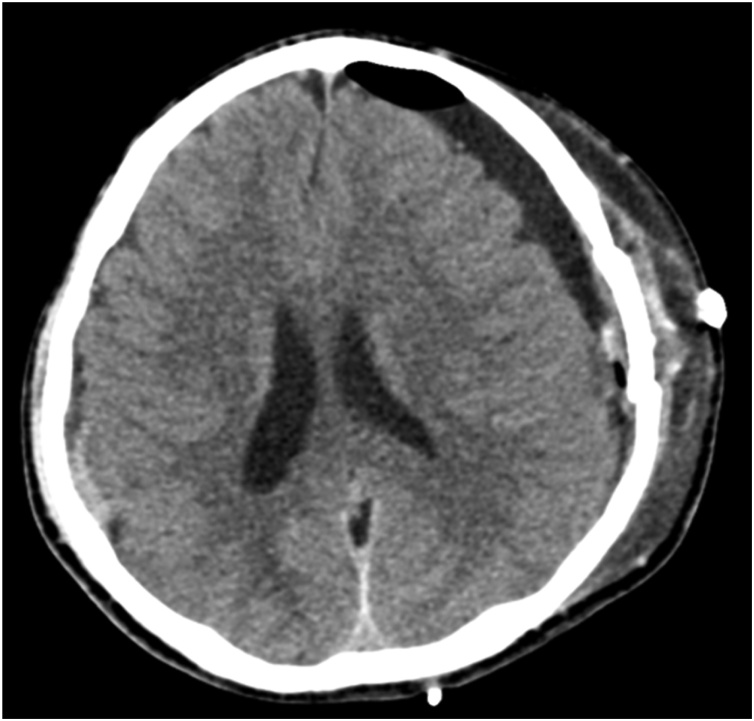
Post-operative CT showing optimum evacuation of left-sided hematoma and a small right-sided SDH.
